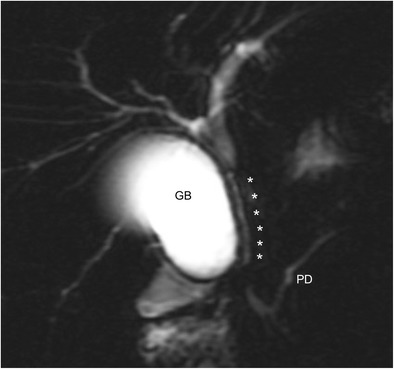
Magnetic resonance cholangiopancreatography (MRCP) showing a dominant stricture. This is a thick-slab heavily T2-weighted MRCP image which shows a long dominant stricture involving the entire length of the common bile duct and the distal common hepatic duct. The dominant stricture is indicated by the *six asterisks* to its *right*. The proximal common hepatic duct just above it and intrahepatic ducts have resultant dilatation. *GB* gall bladder, *PD* pancreatic duct. Figure courtesy of Dr Helen K. Bungay, John Radcliffe Hospital, Oxford, UK
